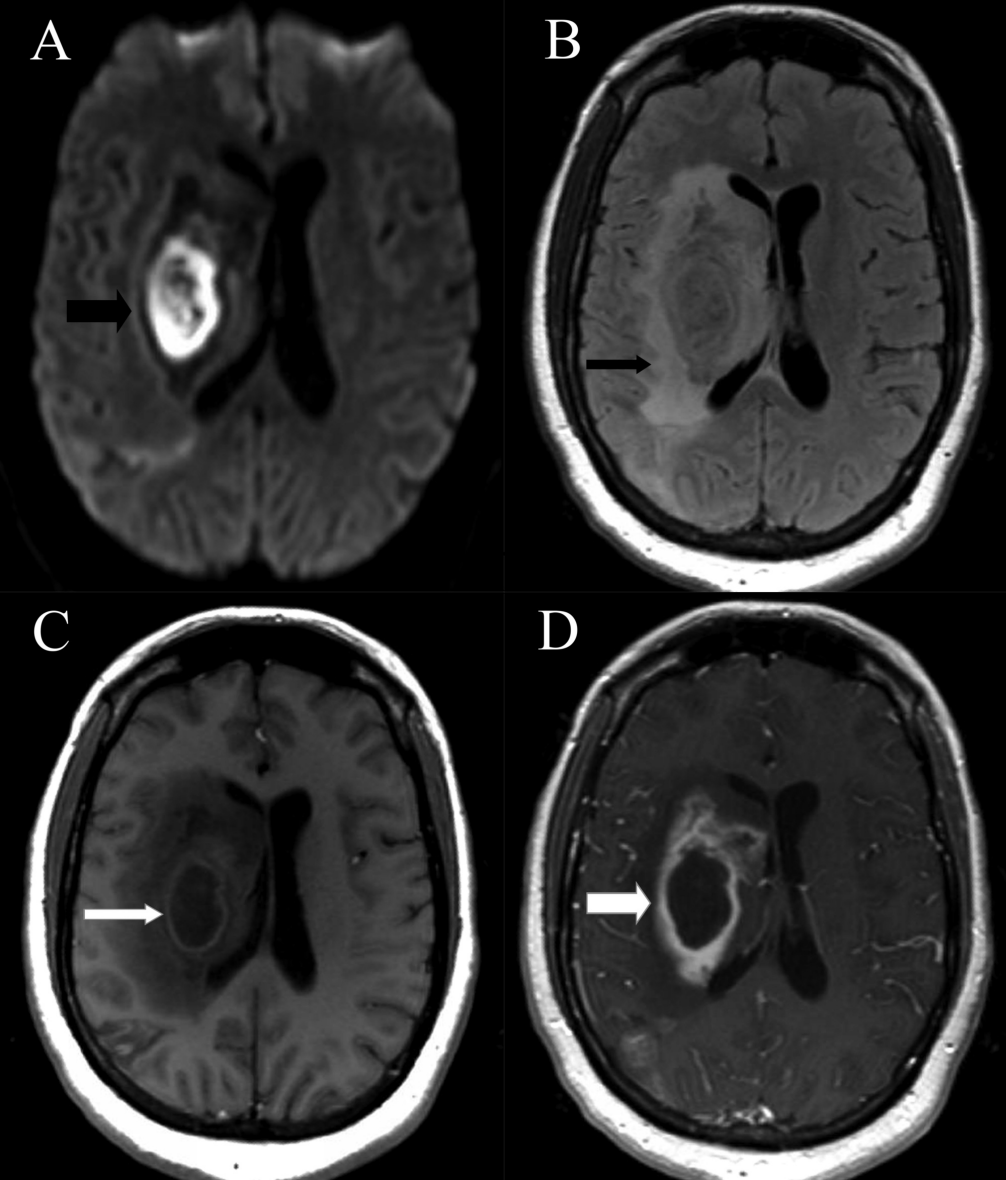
MRI brain after the onset of headache (Axial images) A: DWI sequence with restricted diffusion within the lesion (thick black arrow). B: FLAIR sequence with extensive vasogenic edema around the lesion (thin black arrow). C: T1 hypointensity in the basal ganglia with a thin surrounding wall (thin white arrow). D: T1 post-contrast image with a smooth thin ring enhancement of the lesion (thick white arrow).
